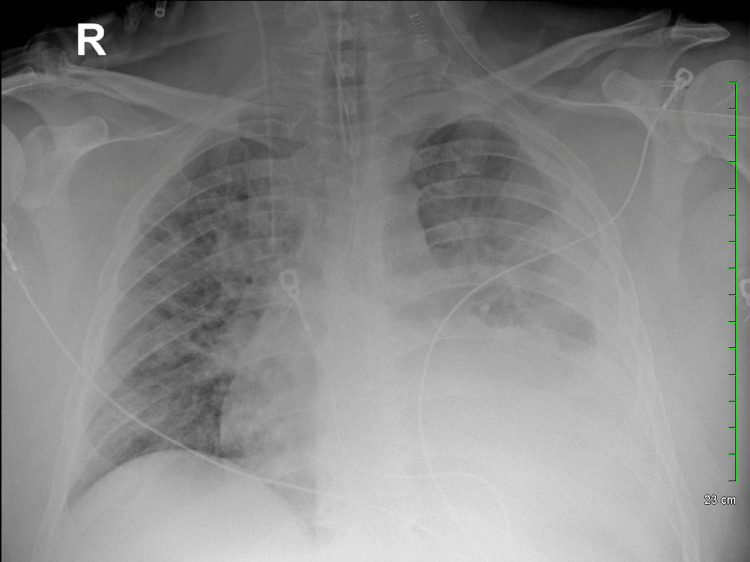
Worsening pleural effusion visualized on X-ray confirming successful endotracheal intubation
